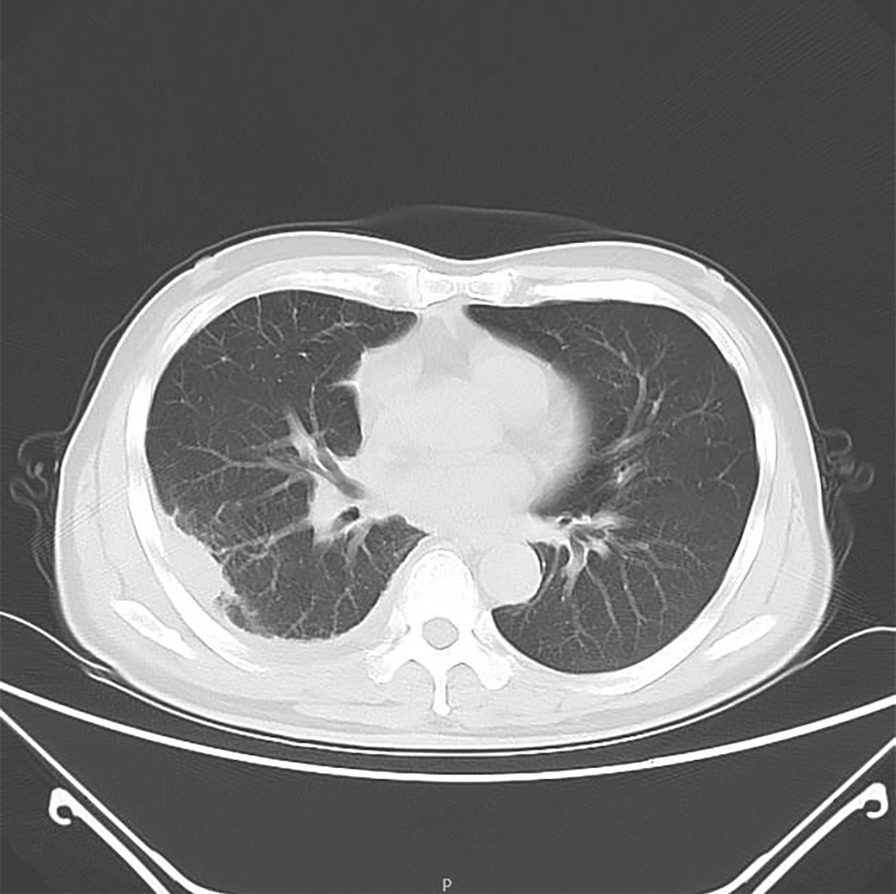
CT of the lung: after treatment, the right pleural effusion was significantly absorbed and the lung tissue was restretched
